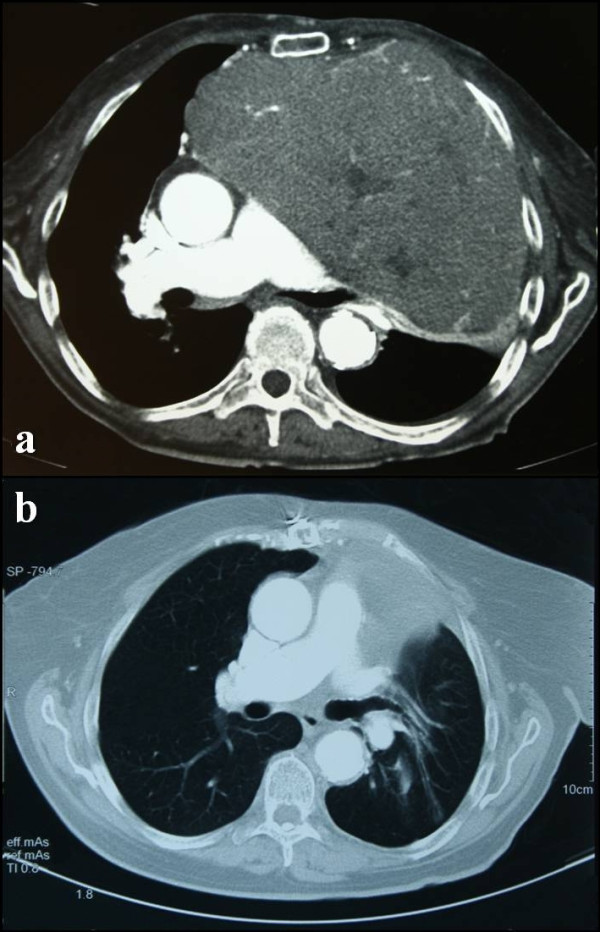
Pre-operative and post-operative chest computed tomography scans. (a) Pre-operative chest computed tomography scan reveals a soft-tissue-density lesion of the anterior mediastinum. (b) Post-operative chest computed tomography scan (same level imaging sections), performed two years after resection of the tumor, shows: no evidence of tumor, the esophagus returned to its anatomical position, decompression of the left main bronchus, expansion of the left lung, correction of the mediastinal width and regain of chest wall subcutaneous fat tissue.
